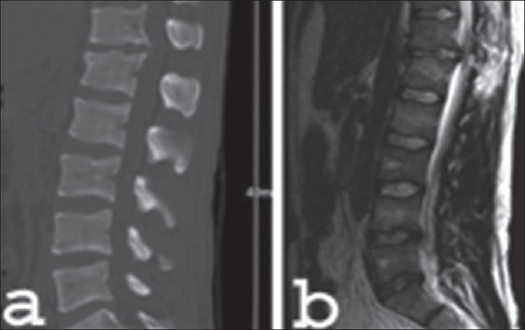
Reconstruction sagittal CT scan (a) and mid sagittal T2WI MRI scan (b) shows flexion destraction type of lesion at D12-L1 with compromise of spinal canal due to disc herniation between T12- L7
